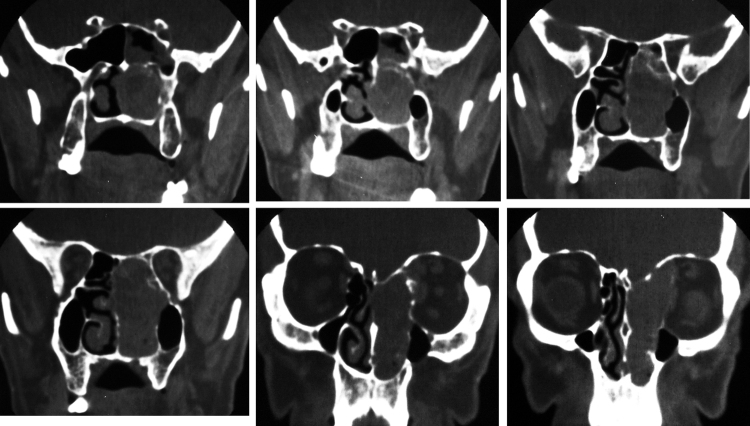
Naso-ethmoid neurofibroma: coronal computed tomography with soft tissue window, showing a naso-ethmoidal solid mass with medial orbital wall remodeling.
